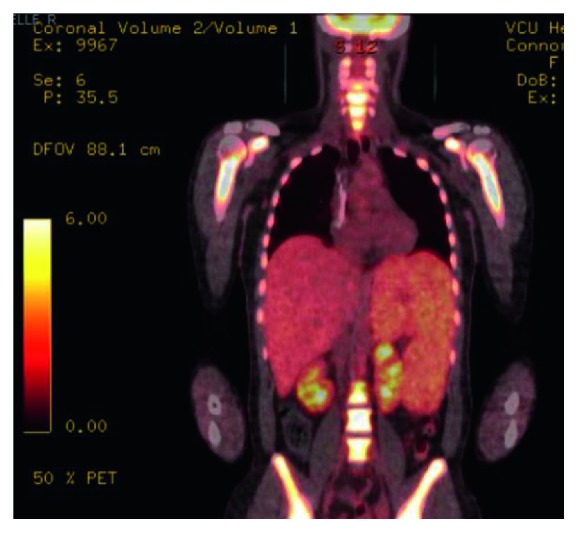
Pet scan 4 weeks after 6 cycles of DA-EPOCH showed massive splenomegaly. The spleen measures up to 27.7 cm in length with pronounced hypermetabolic activity throughout the spleen with a maximal SUV of up to 9.8. There are new peripheral wedge-shaped areas of photopenia consistent with infarcts. There is a pronounced mass effect on the stomach and left kidney from the massive splenomegaly. There is ongoing hepatomegaly as well, with the right hepatic lobe measuring 23.1 cm in length compared to 22.0 previously. Hepatic radiotracer uptake is homogenous but decreased from what would normally be expected (the average maximum SUV is approximately 1.9). This diffusely reduced radiotracer uptake could be secondary to a sequestration of radiotracer in the spleen. The previously described area of low-grade uptake in the left parapharyngeal soft tissues now demonstrates a maximal SUV of 2.0, previously 3.5.
